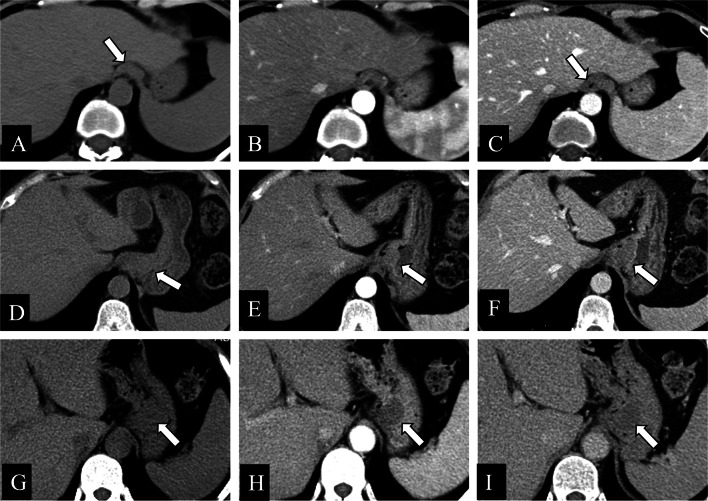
a–c Heterotopic pancreas in a 48-year-old female. Axial contrast-enhanced CT images obtained during the plain (a) /arterial (b) /venous (c) phase showed a flat mass (white arrows) in the gastric cardia, with a tendency to grow horizontally. d–f Gastrointestinal leiomyoma in a 42-year-old male. Axial contrast-enhanced CT images also demonstrated a same flat mass (white arrows) in the gastric cardia, but with a vertical growth tendency compared to the horizontal axis. g–i Gastrointestinal leiomyoma in a 48-year-old female. Axial contrast-enhanced CT images also revealed a vertically growing and round mass (white arrows) in the gastric cardia
